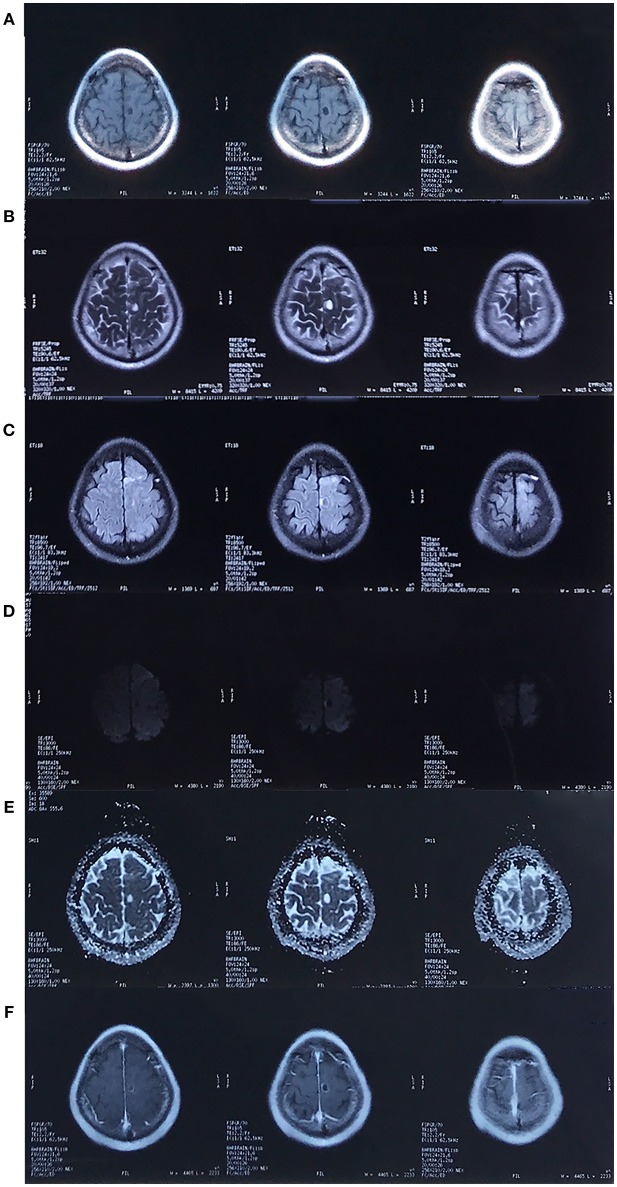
MRI in October 2017. There was a cystic lesion in the left frontal lobe that presented as hypointense on the T1-weighted image (A), hyperintense on the T2/T2-FLAIR/Apparent diffusion coefficient (ADC) image (B,C,E) and hypointense on the diffusion weighted imaging (DWI, D), with surrounding edema and rim enhancement (F).
